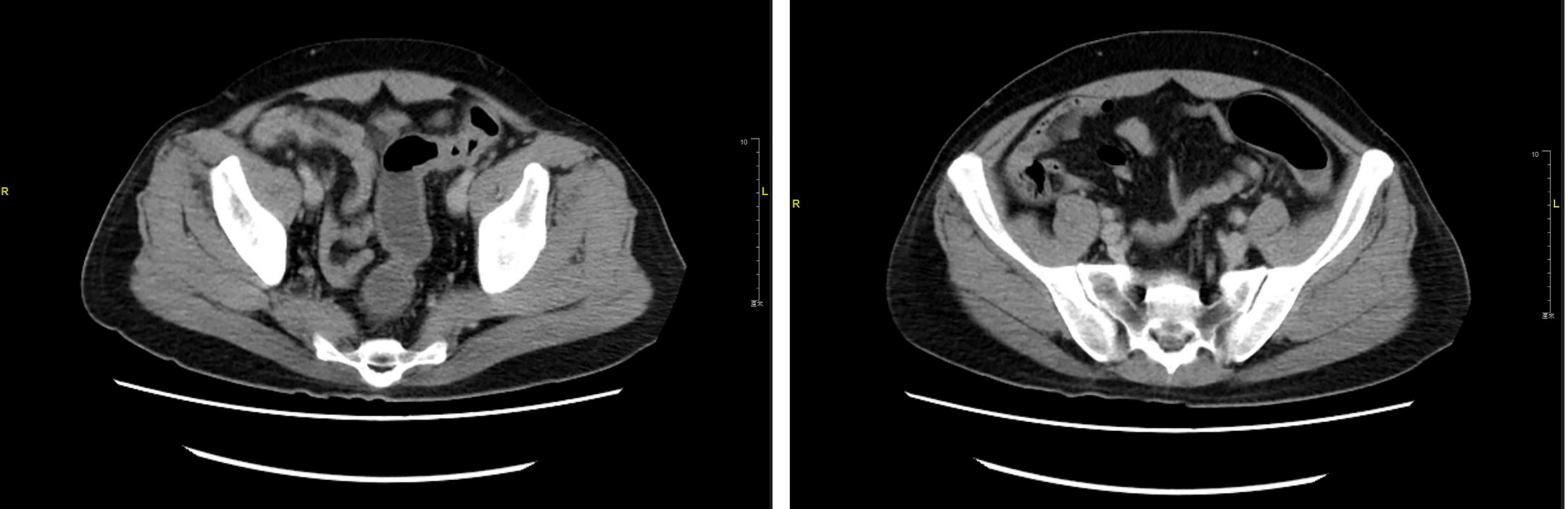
CT manifestation after recurrence.
